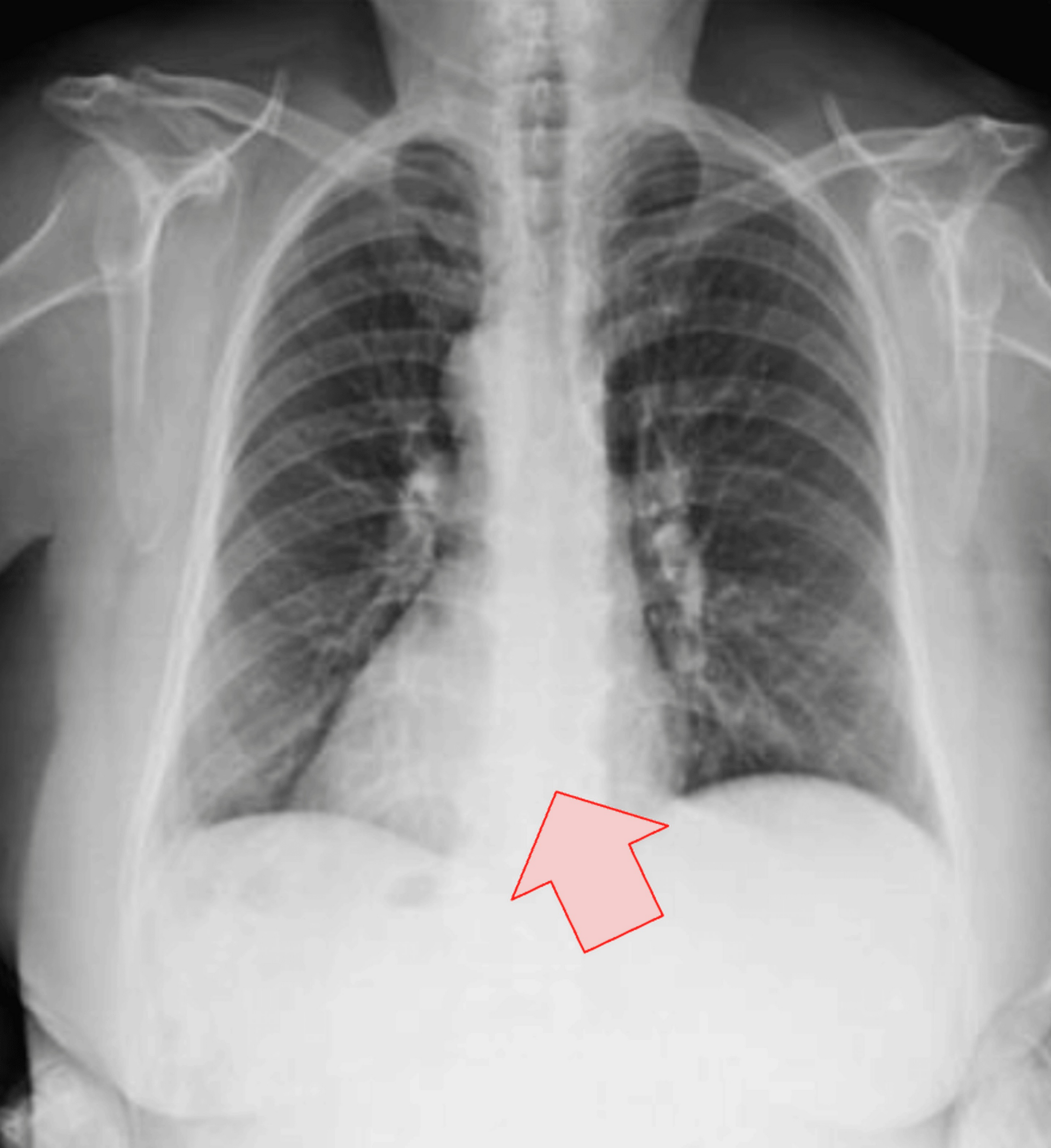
Chest radiograph demonstrating dextrocardia Posteroanterior chest radiograph showing the cardiac apex positioned on the right hemithorax (arrow), consistent with dextrocardia and suggestive of situs inversus totalis in the appropriate clinical context.
